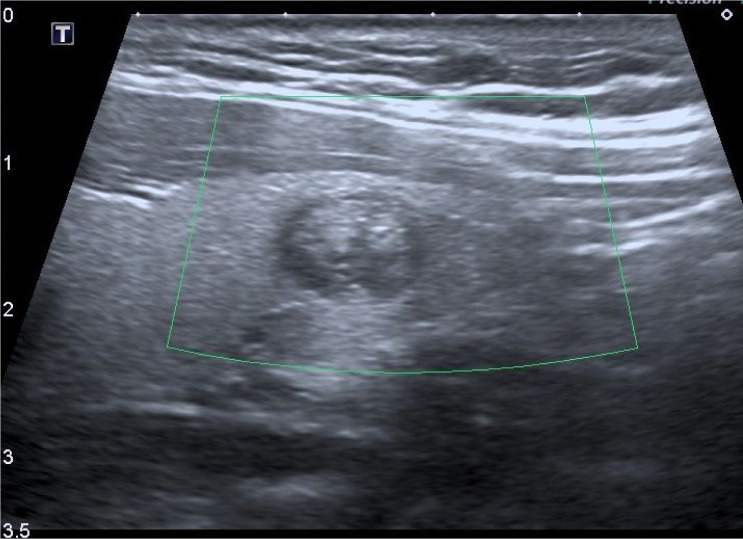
Solid nodule without any vascularization on color Doppler examination, located at the lower pole, right lobe. The pathological diagnosis is a benign colloid nodule.
